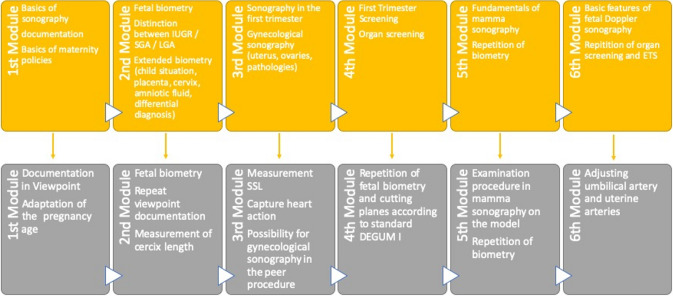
The different learning goals of the comprehensive ultrasound curriculum
